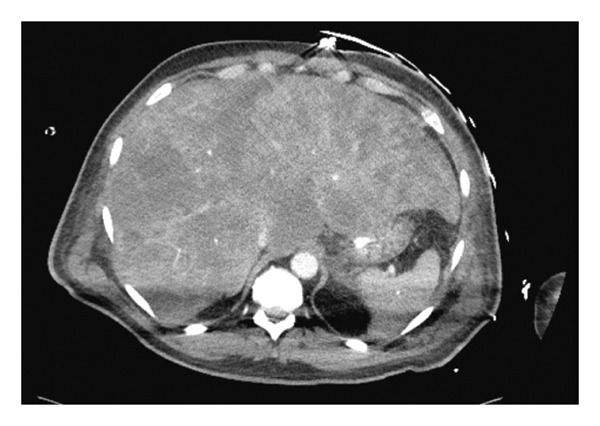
Abdominal CT scan of HLH patient with apparent hepatomegaly.
